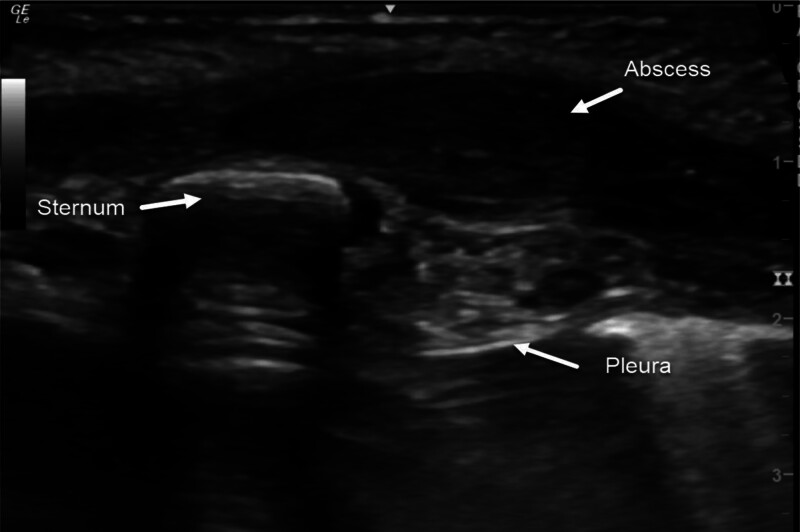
Axial ultrasound showing hypoechogenic mass presternal, suggesting abscess formation with tissue alteration reaching the retrosternal space.
